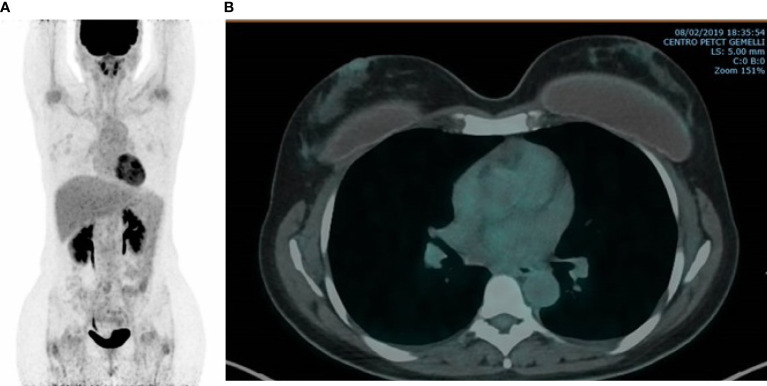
FDG-PET/CT. Completely disappeared bone (A) and breast (B) uptake was observed after five months of AI, LH-RHa and CDK4/6 inhibitor.
